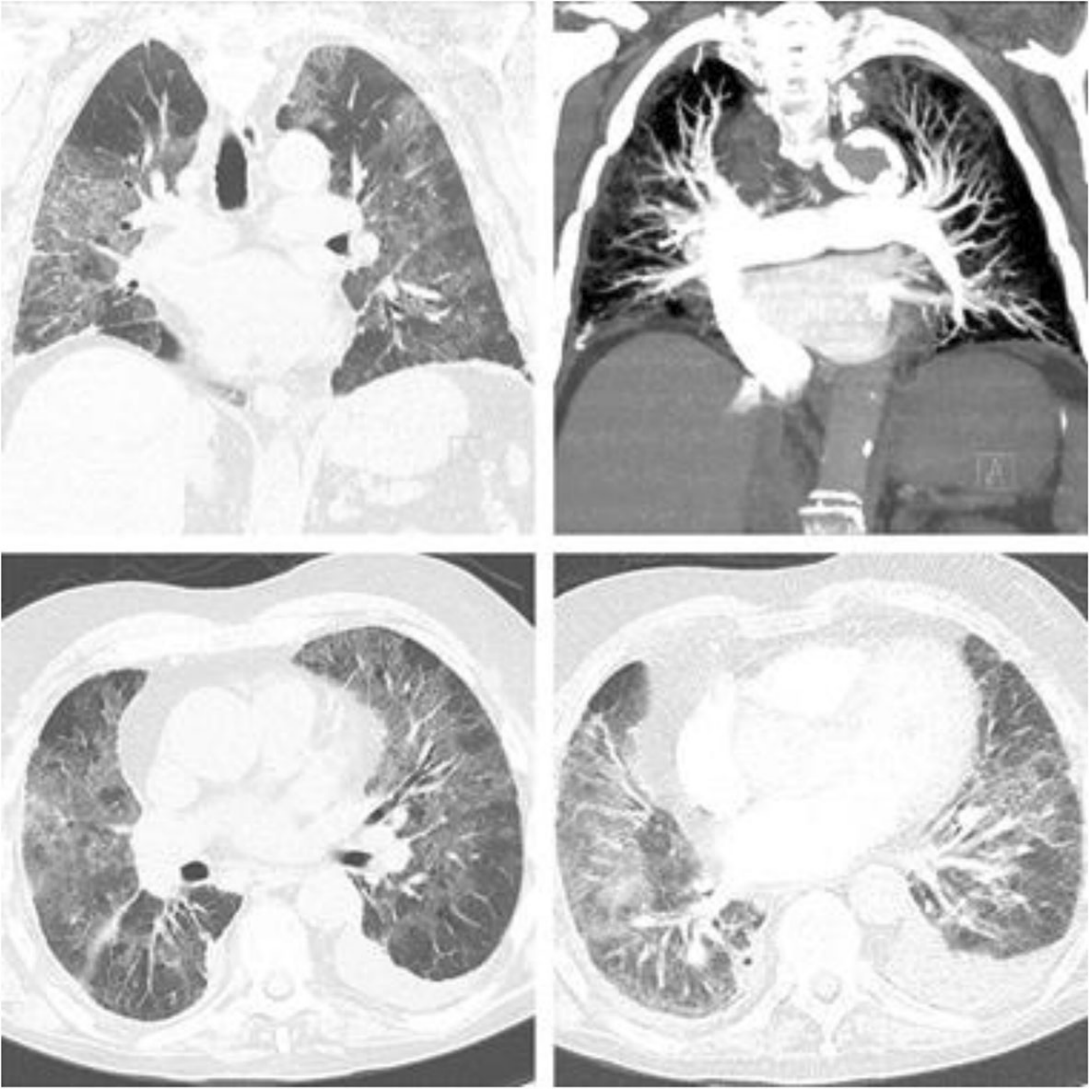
Thorax CT-Scan showed multiple areas of ground-glass opacification
